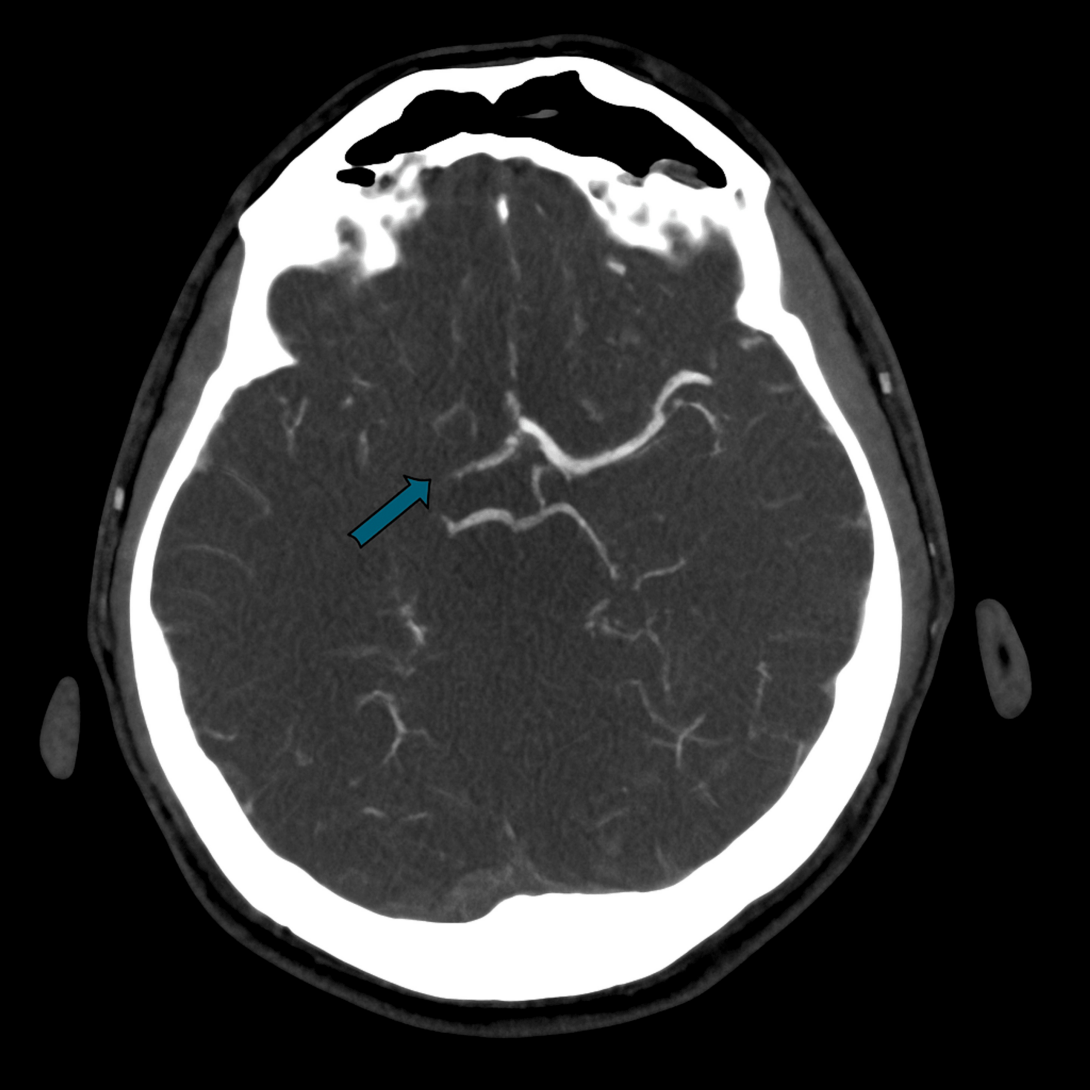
CT angiogram showing right middle cerebral artery occlusion (outlined in green).
